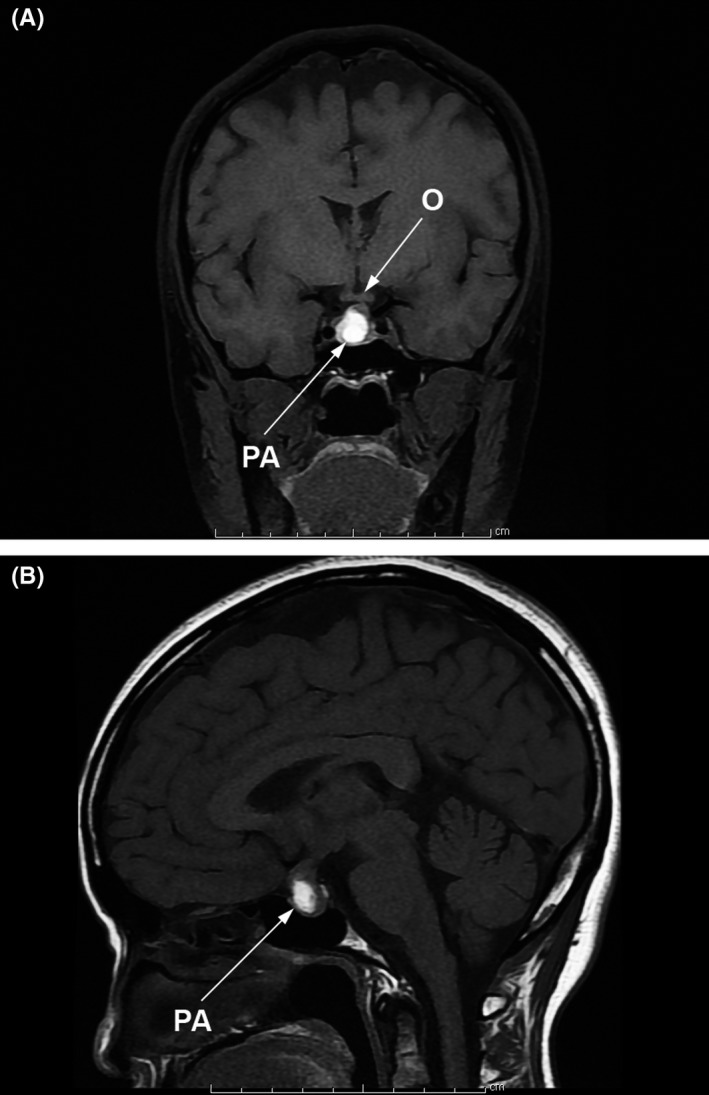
T1‐MRI Pituitary coronal (A) and sagittal (B) scan at 16 wk of gestation depicting pituitary apoplexy (PA) and minimal compression of optic chiasm (O)
